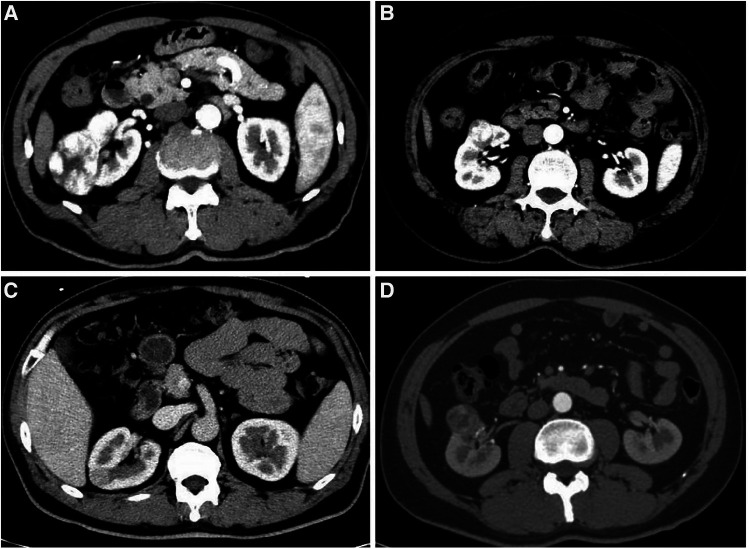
The images represent actual cases of offc-RAPN. (A) preoperative CT depicting the largest diameter of a large and exophytic rate >50% tumor within the renal parenchyma <4 cm; (B) preoperative CT showing the largest diameter of an exophytic rate <50% tumor within the renal parenchyma <2 cm; (C) preoperative CT revealing the largest diameter of an endophytic tumor <2 cm; (D) preoperative CT presenting the largest diameter of an exophytic rate >50% and hilar tumor <4 cm.
